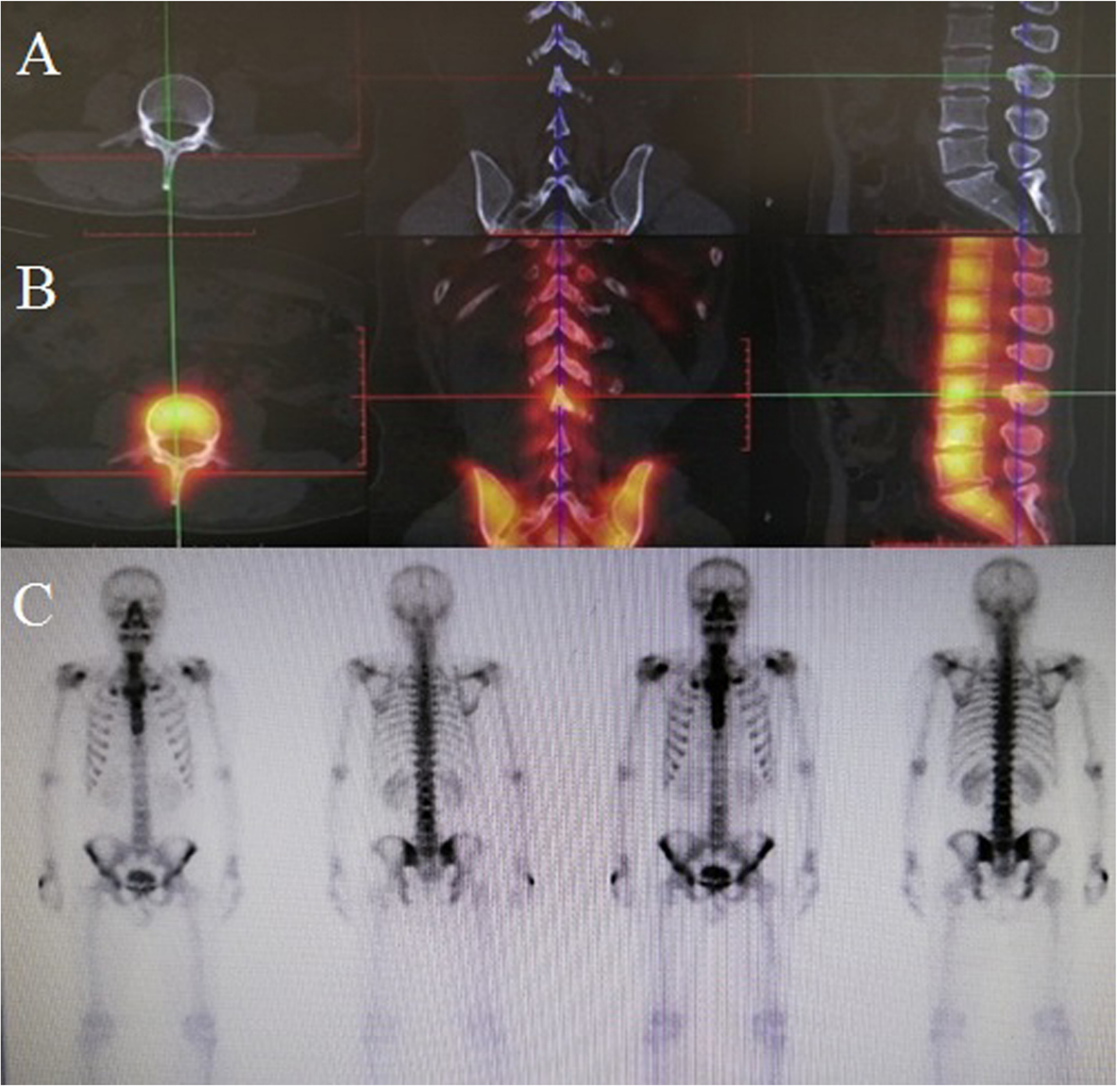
Preoperative whole body bone scan with single photon emission computed tomography (SPECT). SPECT/CT did not show bone destruction (a). Abnormal ^99m^Tc-methylene diphosphonate (MDP) accumulation was demonstrated in the L3 lamina and spinous process on the fused SPECT/CT (b) and whole body bone scan (c). No signs of other bone destruction and metastasis were observed in the examination
